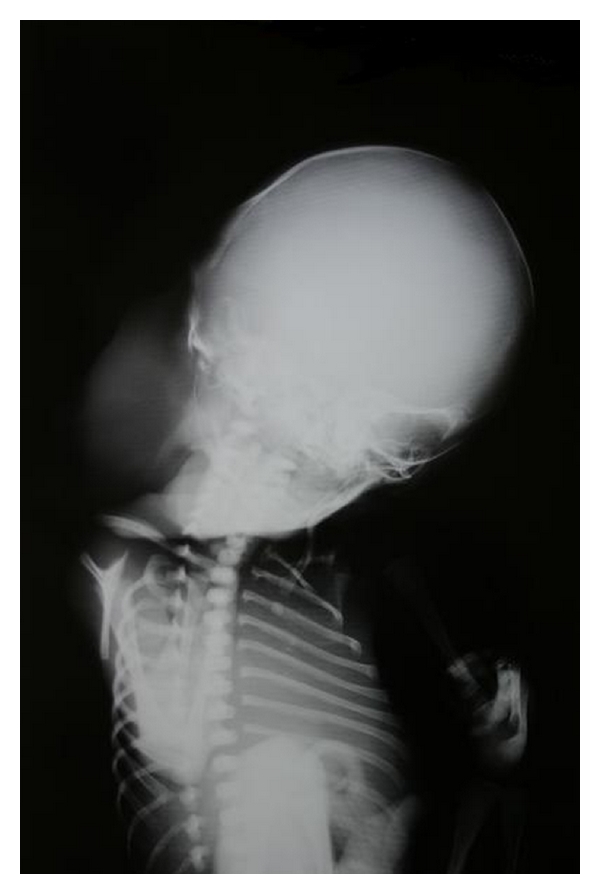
X-ray of the aborted fetus.
